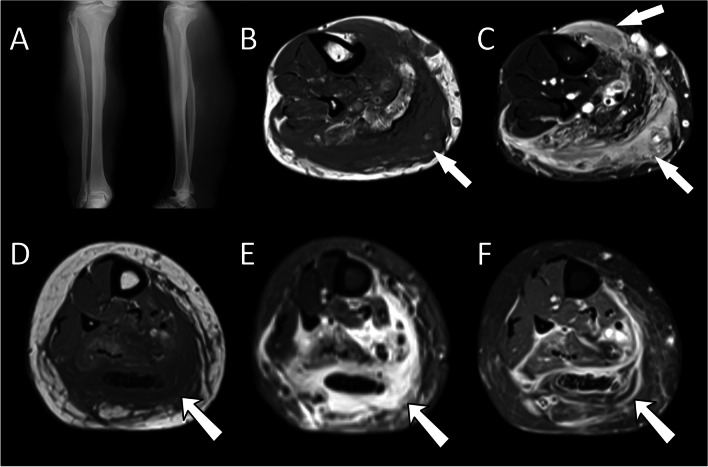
A Anteroposterior and lateral radiographs of the tibia and fibula in a patient with neurofibromatosis type 1 presenting with an enlarged calf comprising a diffuse plexiform neurofibroma and dysplasia of both the tibia and fibula. Axial T1W (A) and T2 fat-saturated (B) images through the calf reveal a diffuse seemingly “infiltrative” intermediate T1w and hyperintense T2W soft tissue lesion that does not respect anatomical compartments (arrows). Biopsy of the lesion demonstrated a diffuse plexiform neurofibroma that remained stable on MR imaging over a period of 12 years
